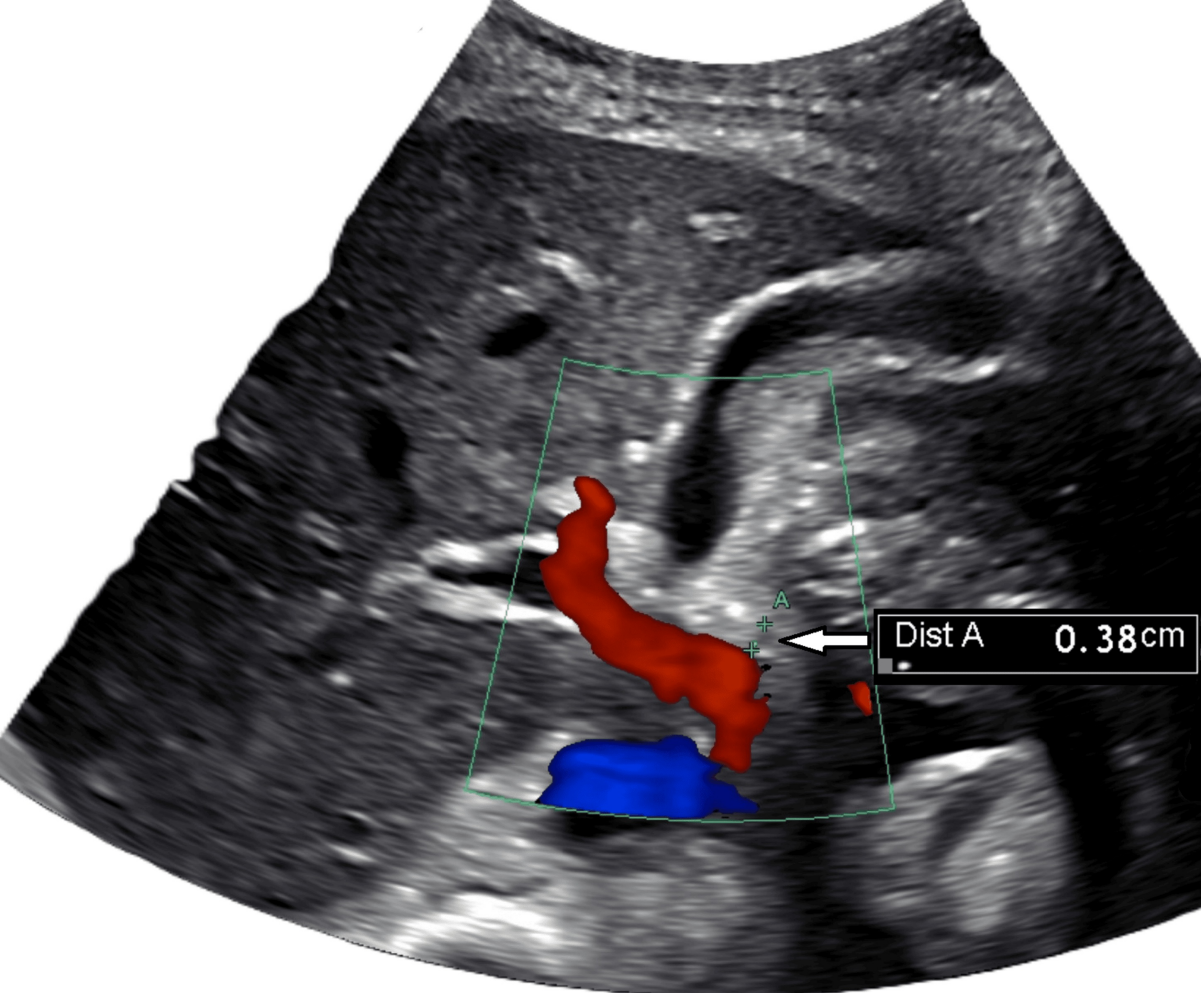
Right upper quadrant abdomen ultrasound of the common bile duct The right upper quadrant abdomen ultrasound shows a common bile duct (arrow) measuring 3.8 mm without dilatation or stones.
